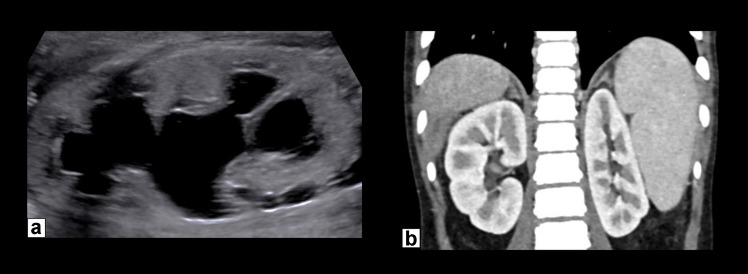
a Representative sagittal US image of the right kidney demonstrating hydroureteronephrosis at birth in a female patient with MMIHS. (b) Coronal CT abdomen/pelvis with IV contrast at age 5 shows normal kidneys without hydronephrosis. This patient was initially managed via bladder catheterization but now spontaneously voids three to four times per day
